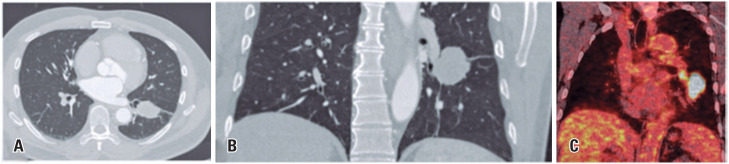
Coronal and axial views of coronary angiotomography with pulmonary window (A and B) revealed a lung mass located in the left lower lobe. The mass showed contact with the origin of the basal segment bronchi, resulting in mild subsegmental bronchi obliteration. Furthermore, the PET-CT scan reformatted image (C) showed intense glycolytic hypermetabolism (SUVmax 26.5) within this lesion, indicating a high likelihood of a primary neoplasm, and a suspected lymph node (SUVmax 6.9) involvement
